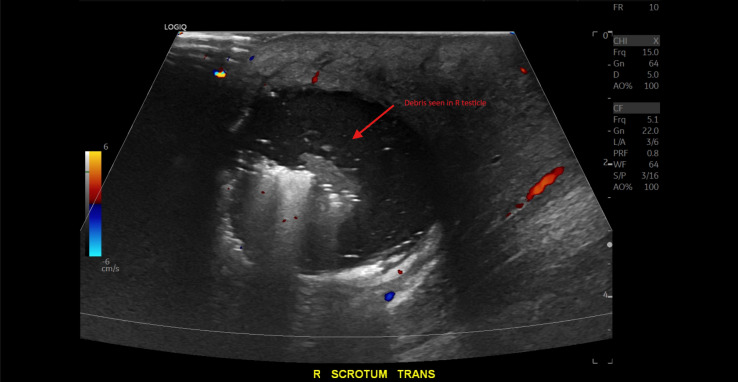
Point of care ultrasound (POCUS) (transverse view) of the right side of the scrotum shows shadowing and a debris pattern consistent with gas in the right testicle. R, right; Trans, transverse.
